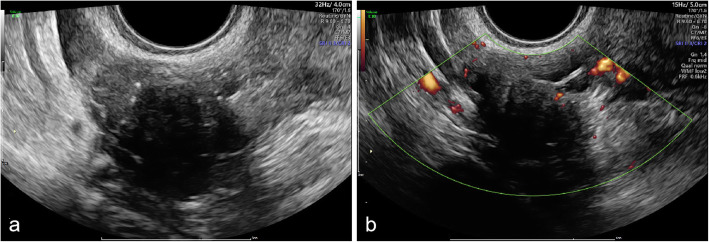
a hypoechoic mass was observed in the right ovary with posterior echo attenuation by two-dimension ultrasound examination, confirmed thecoma-fibroma finally; b Energy Doppler showed the hypoechoic mass was with minimal Doppler signals
